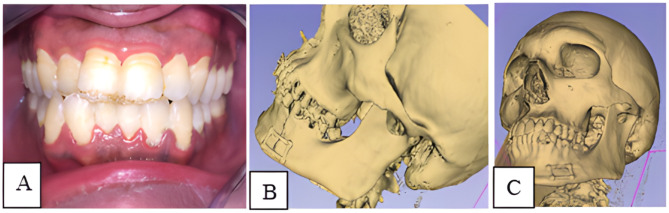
(A) Postoperative occlusion. (B) Immediate postoperative CT reconstruction. (C) Postoperative CT reconstruction after 3 months
